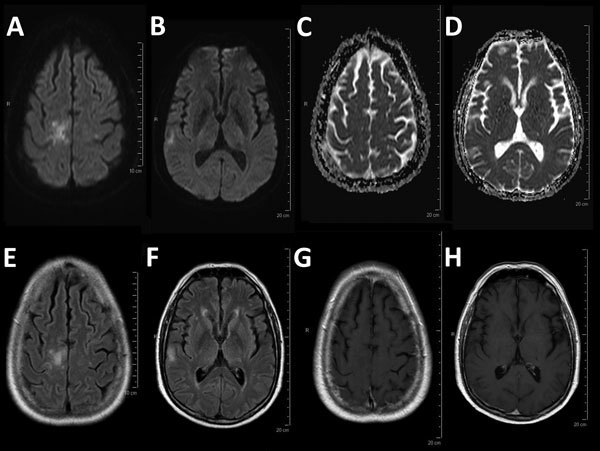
Magnetic resonance imaging for a 54-year-old man with progressive multifocal leukoencephalopathy after treatment with nivolumab, showing typical multifocal lesions: diffusion weighted imaging hyperintensity (A, B) with no decrease in the apparent diffusion coefficient (C, D), corresponding patchy corticosubcortical hyperintensities on fluid-attenuated inversion recovery image (E, F) without enhancement on T1-weighted imaging after administration of gadolinium (G, H).
